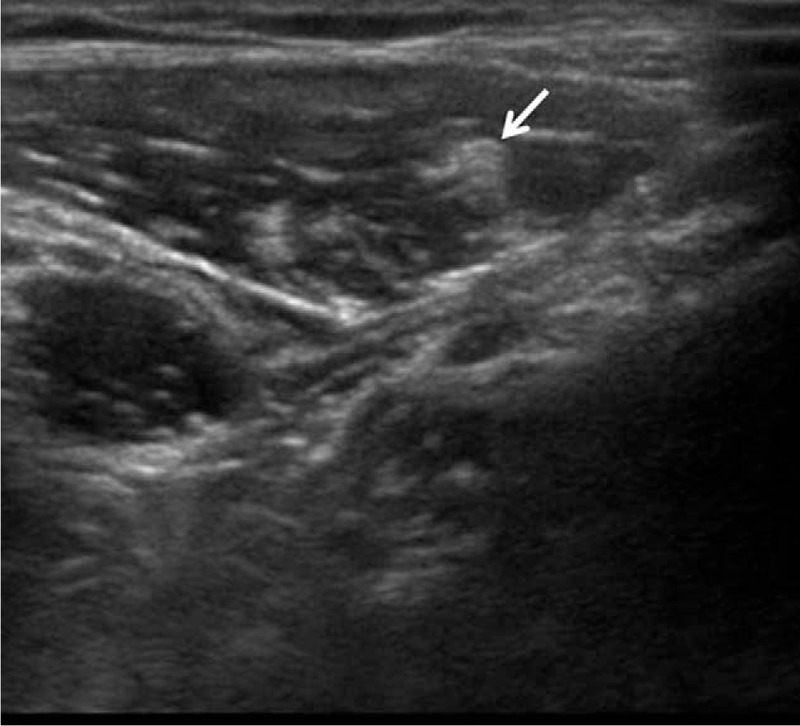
Ultrasound image of the lateral femoral cutaneous nerve (arrow).
